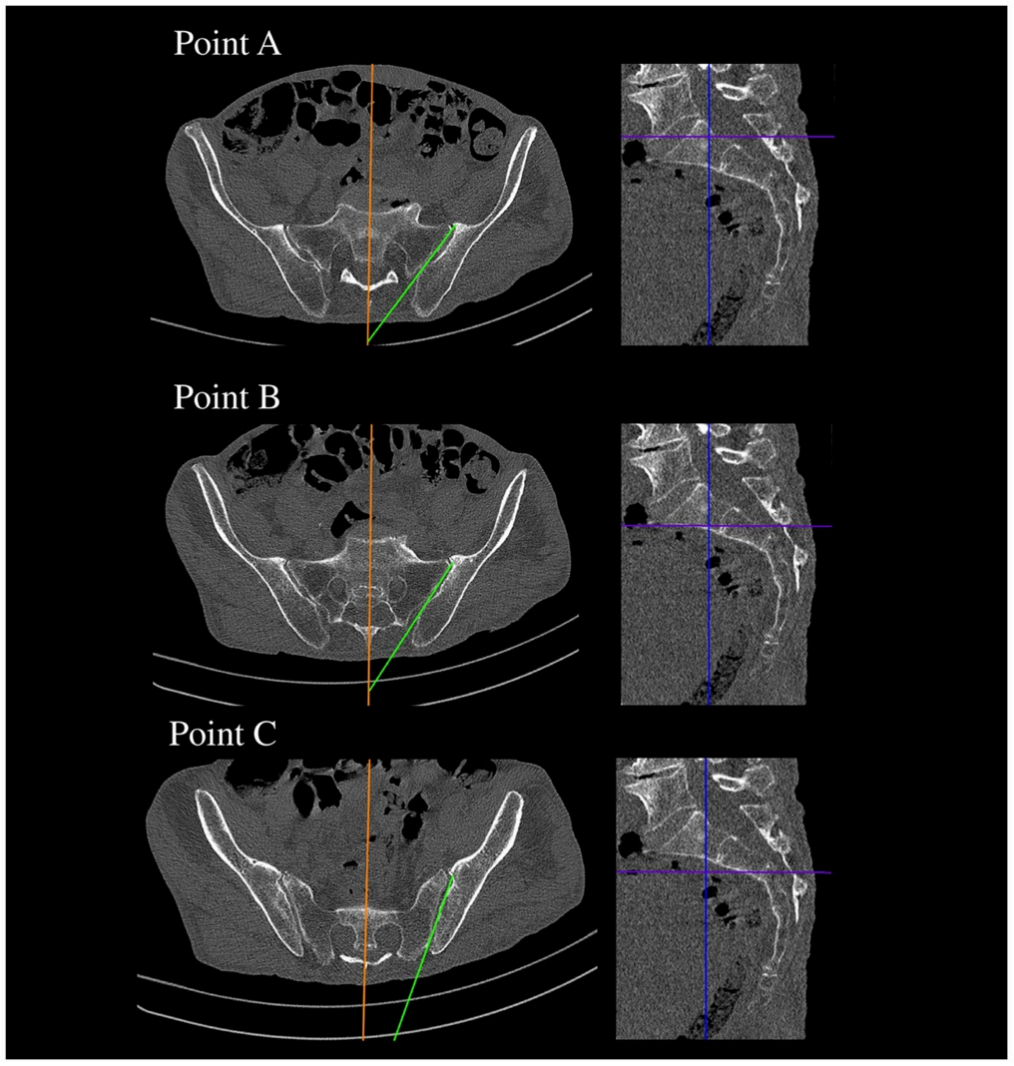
CT showing the angle between the median plane and SIJ—This measurement corresponds to the angle, at which a screw should be directed.
